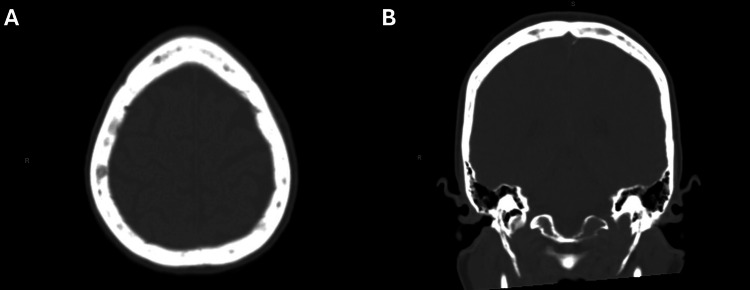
CT of the head - axial and coronal views A (axial view) and B (coronal view) demonstrate multifocal, nonspecific osseous lucencies within the right supraorbital rim and calvarium, concerning for osseous metastases or myelomatous involvement. Additional findings include age-related cerebral volume loss and mild paranasal sinus disease. CT, computed tomography
